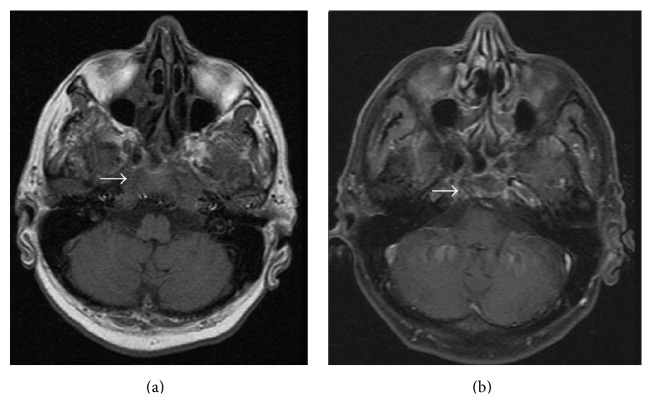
Initial axial enhanced T1-weighted MRI (a) show enhancement (arrow) of the clivus and occipital condyles corresponding to infiltration. Eight months later after systemic therapy axial enhanced T1-weighted MRI (b) shows significant reduction of the enhancement (arrow).
